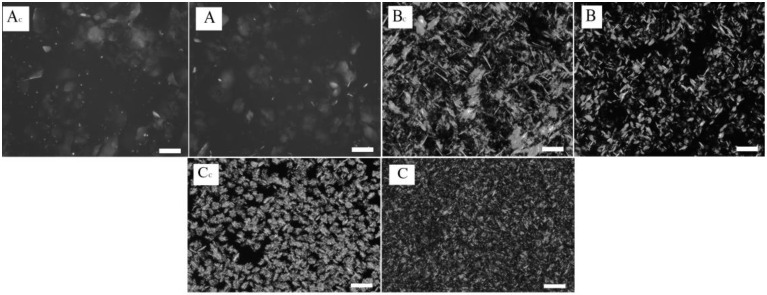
Microstructure of oleogels contained different fractions of beeswax treated and untreated by ultrasound when obtained. (Ac,Bc,Cc)—oleogels without ultrasonic treatment. (A,B,C)—oleogels with ultrasonic treatment. (Scale 100 µm, “c” is the coefficient indicating the control sample).
